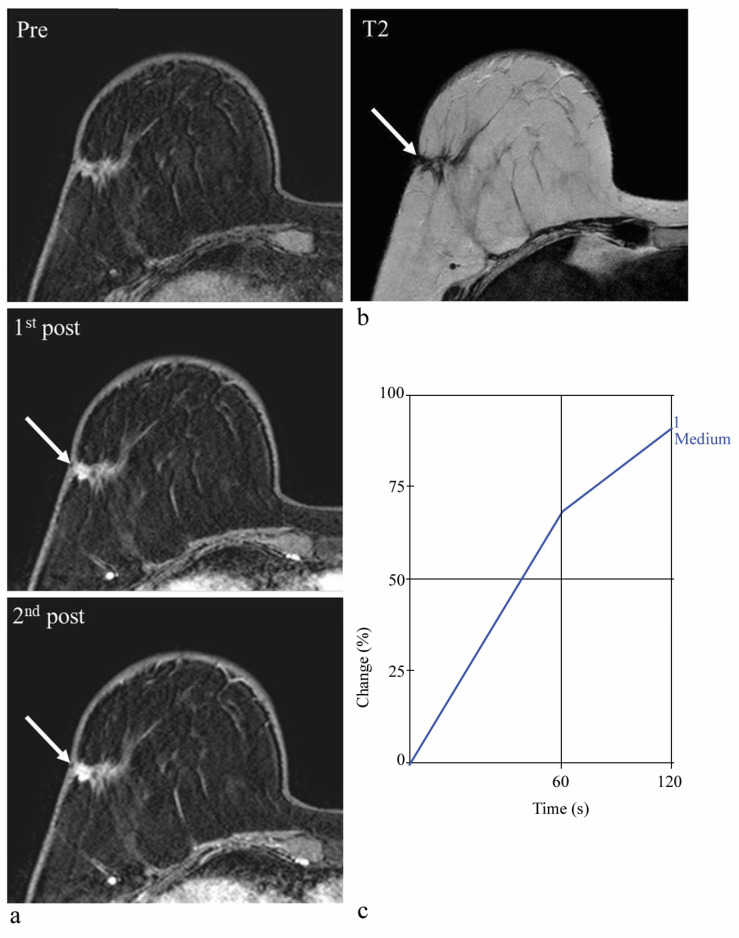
Screening abbreviated breast MRI (AB-MRI) images of a 67-year-old woman with a history of right breast-conserving surgery due to breast cancer. Four years after surgery, pre-contrast and two post-contrast-enhanced axial images (a) show a 1.1 cm irregular enhancing mass (arrows) in the right breast at the operation site. The T2 signal intensity is not high (b). This lesion was assessed as BI-RADS 4C category in the original report. According to the interpretation guideline, it is classified as a suspicious lesion for which biopsy is recommended. However, kinetic analysis shows a low enhancement degree of 68% on the first post-contrast-enhanced image (c). This lesion was detected by second-look ultrasound (US) and confirmed as invasive ductal carcinoma by US-guided core biopsy and subsequent surgery. This is a false-negative case when the enhancement degree on the first post-contrast-enhanced image is combined with morphological analysis.
